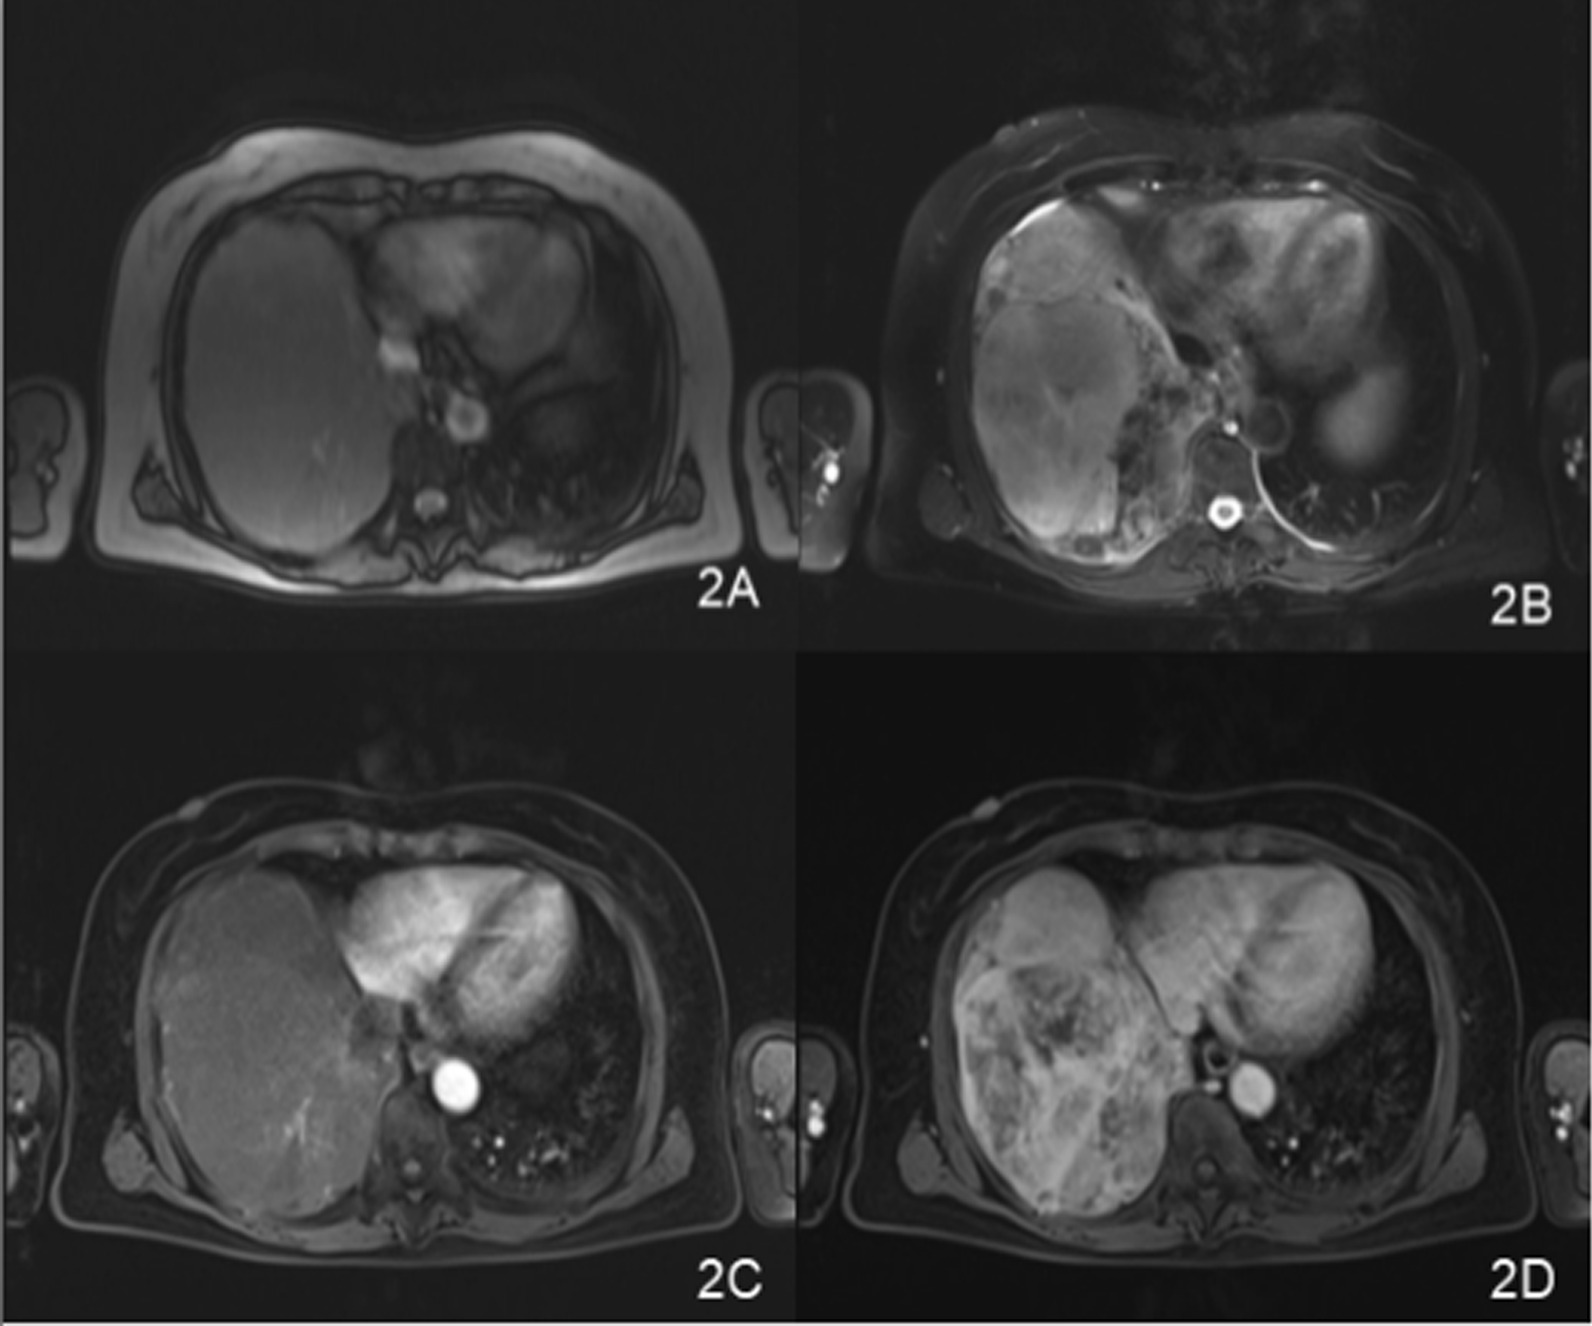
Chest MRI examination showing A low signal intensity on T1WI, B slightly higher signal intensity on T2WI, and low signal intensity on the internal strip. Contrast-enhanced MR demonstrates obvious heterogeneous enhancement of the lesion, and the necrotic area is not enhanced (C, D)
